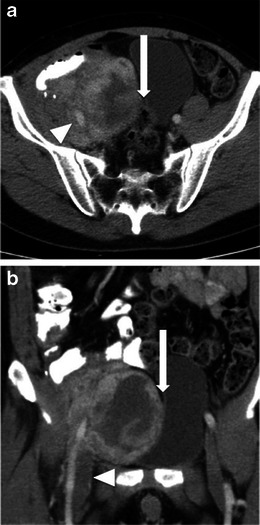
Imaging of MFH. A 57-year-old man who presented with abdominal pain. a Axial contrast enhanced CT shows a large right-sided heterogeneous mass with central low attenuation in keeping with necrosis. The mass is displacing local structures, including bladder and bowel loops (*arrow*). The right external iliac artery can be seen coursing through the mass (*arrowhead*). b Coronal reconstruction shows the extent of the mass and displacement of the bladder (*arrow*). There is thrombus within the right common femoral vein (*arrowhead*)
